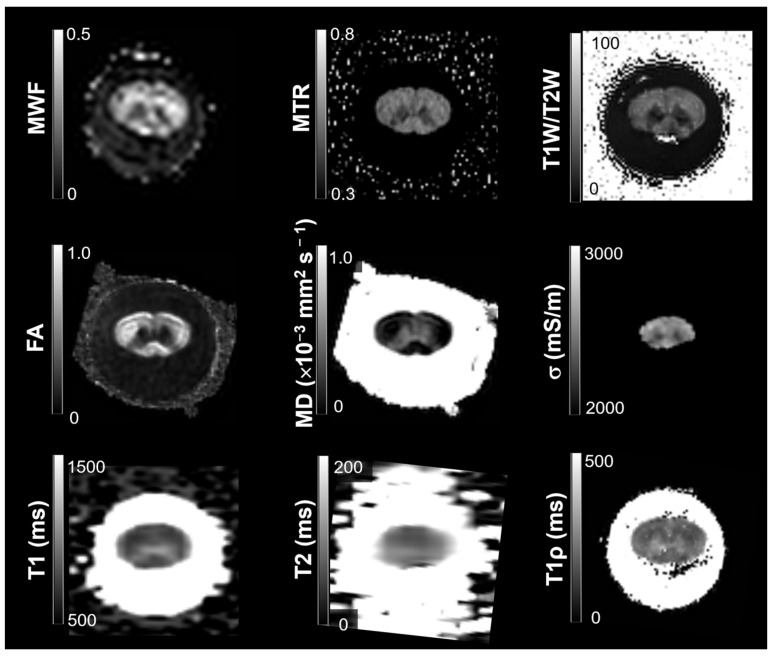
Representative co-registered maps of the quantitative MRI indices. The name of each index is given on the left side, along with the look-up table.
